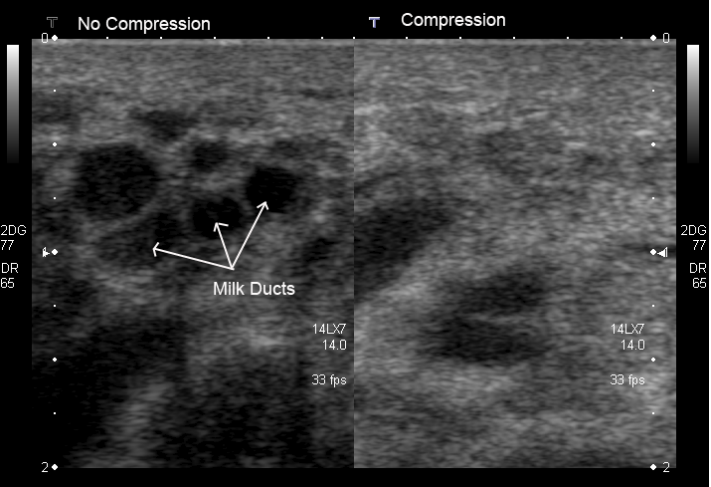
Cross-sectional ultrasound image of milk ducts in the lactating breast. On the left image, milk ducts appear as oval hypoechoic (black) structures. On the right image, milk ducts have collapsed under minimal to moderate compression with the ultrasound transducer.
